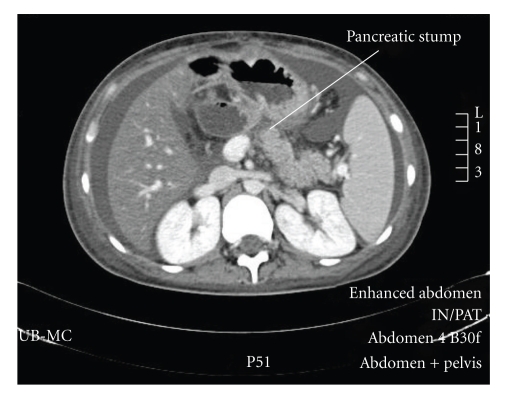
Abdominal CT scan showing the disconnected pancreatic stump with fluid in the abdomen.
